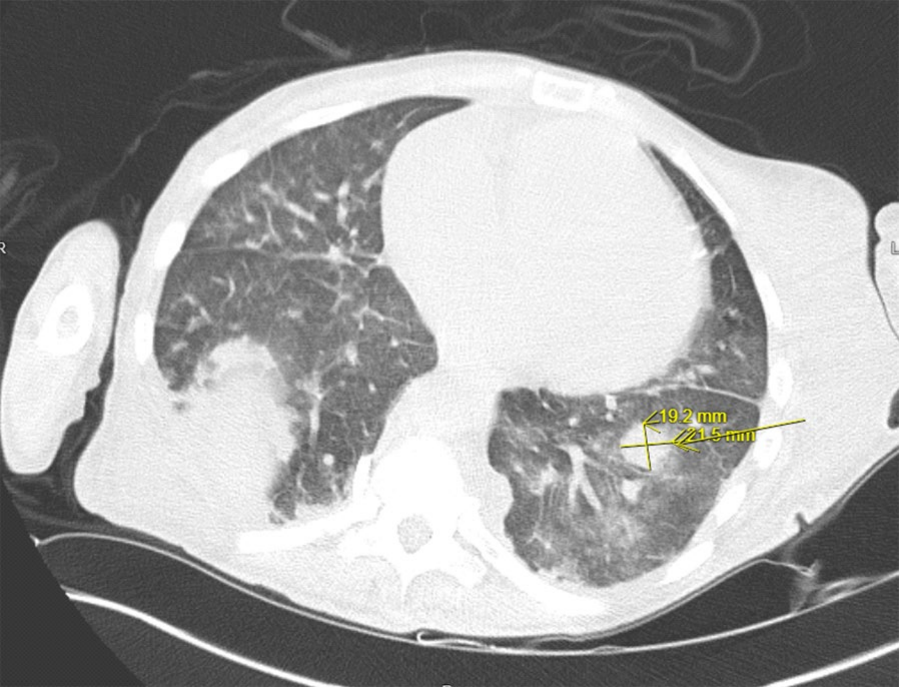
CT chest, abdomen, pelvis with coalescing regions of opacities and nodular density within the left lower lobe
